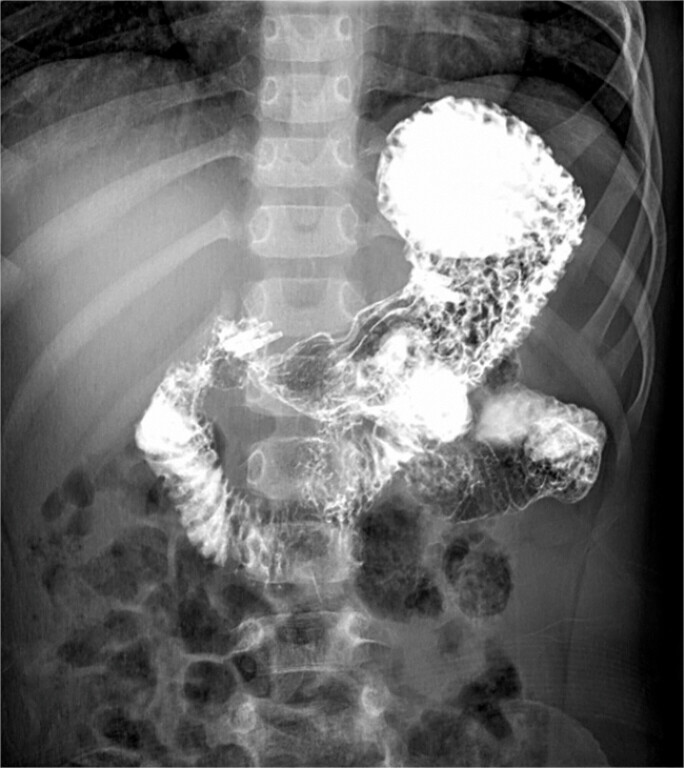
Postoperative upper gastrointestinal tract radiography at one month shows complete healing of the fistula, with no passage of contrast agent through the fistula into the duodenum.
